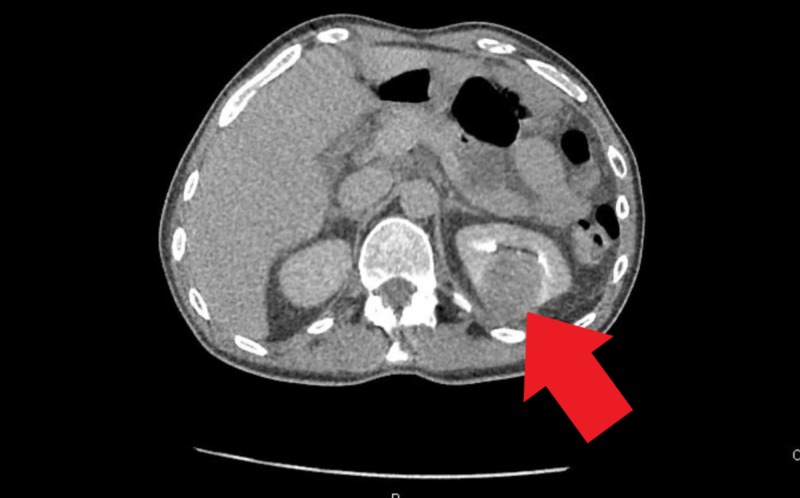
Left renal abscess as noted on the computed tomography scan
